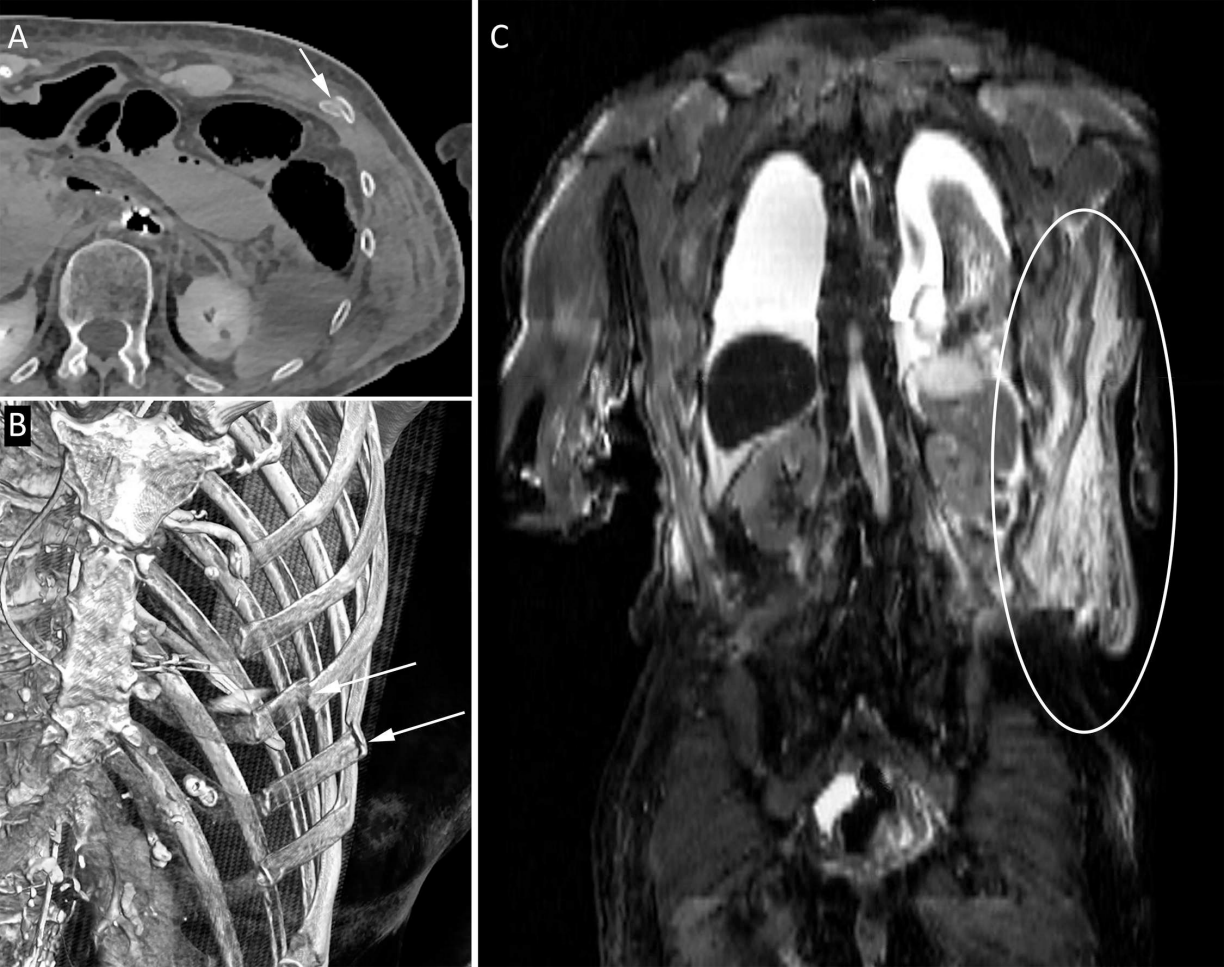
Rib fractures with soft-tissue hematoma. 89-year-old woman who underwent an elective coronary angiography for mitral valve insufficiency and complaints of angina. During the procedure she developed cardiac arrest and required cardiopulmonary resuscitation. She was transported to the intensive care, where she showed symptoms of hypovolemic shock and she died shortly afterwards. Postmortem CT showed multiple rib fractures (A and B: arrows) and on postmortem MRI a large soft-tissue hematoma was seen in the left flank (C: ellipse).
